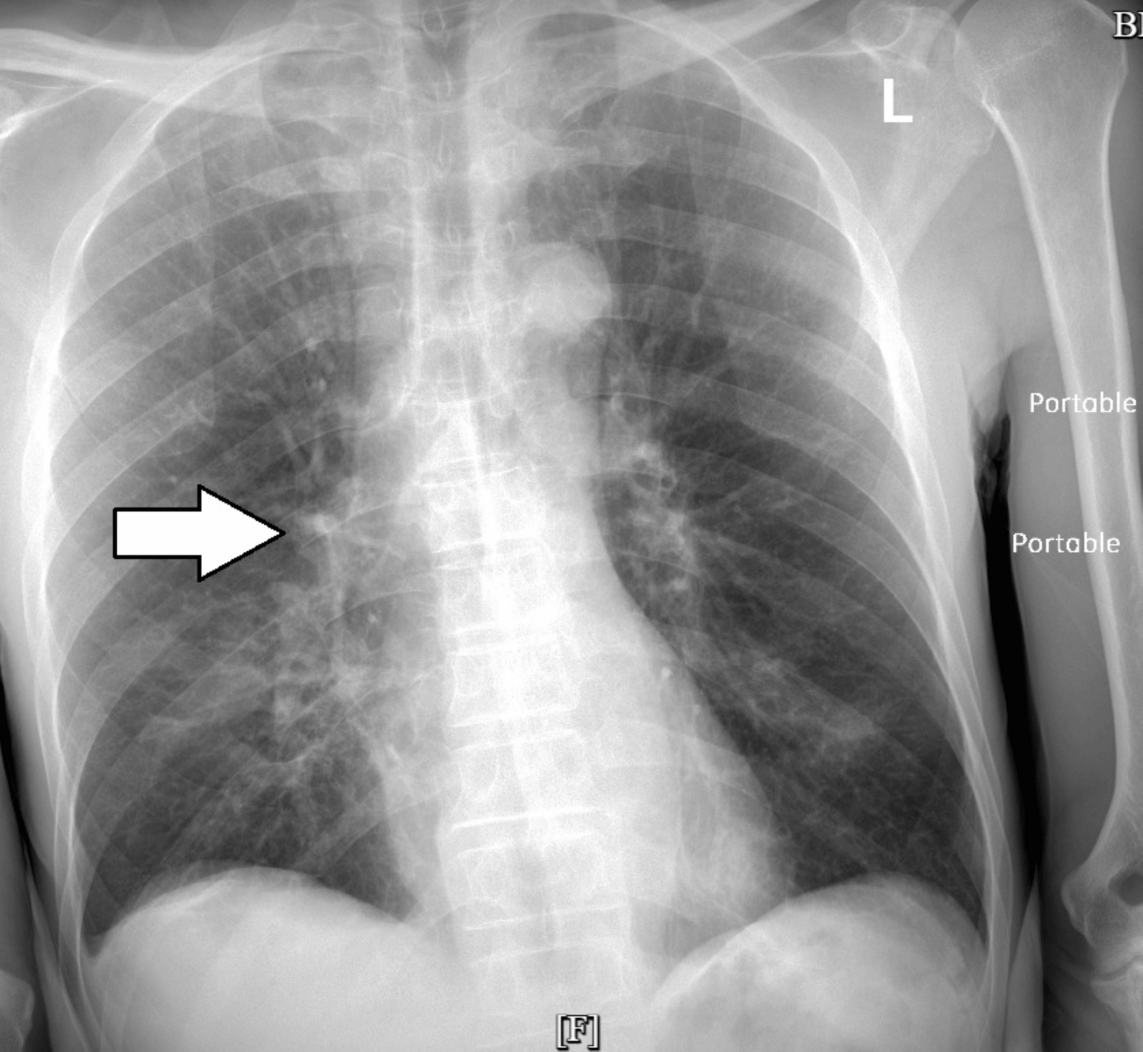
Chest X-ray showing patchy airspace opacity in the perihilar right lung (white arrow)
